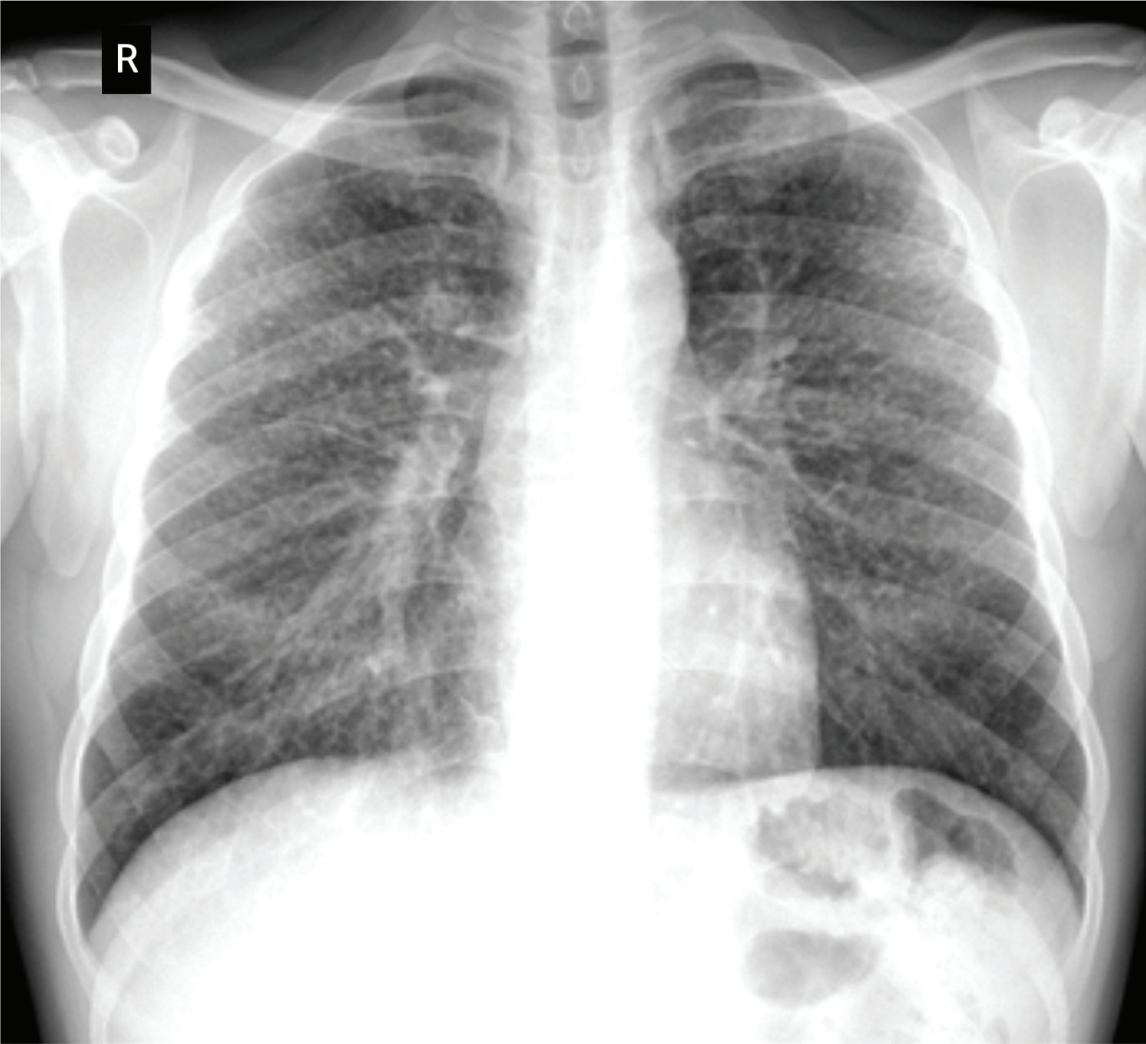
Posteroanterior chest radiograph.
